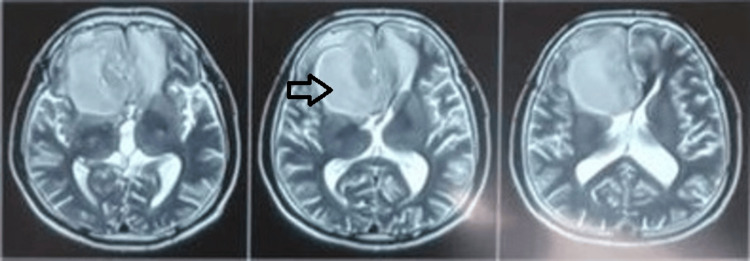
Depicting pre-operative MRI brain (T2 images); heterointense intra-axial space-occupying lesion in the right frontal lobe.
